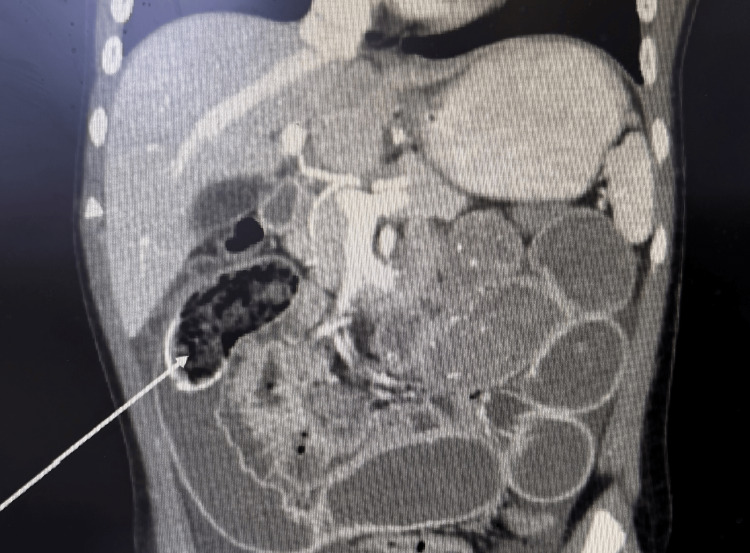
CT abdomen showing the trichobezoar (white arrow)
